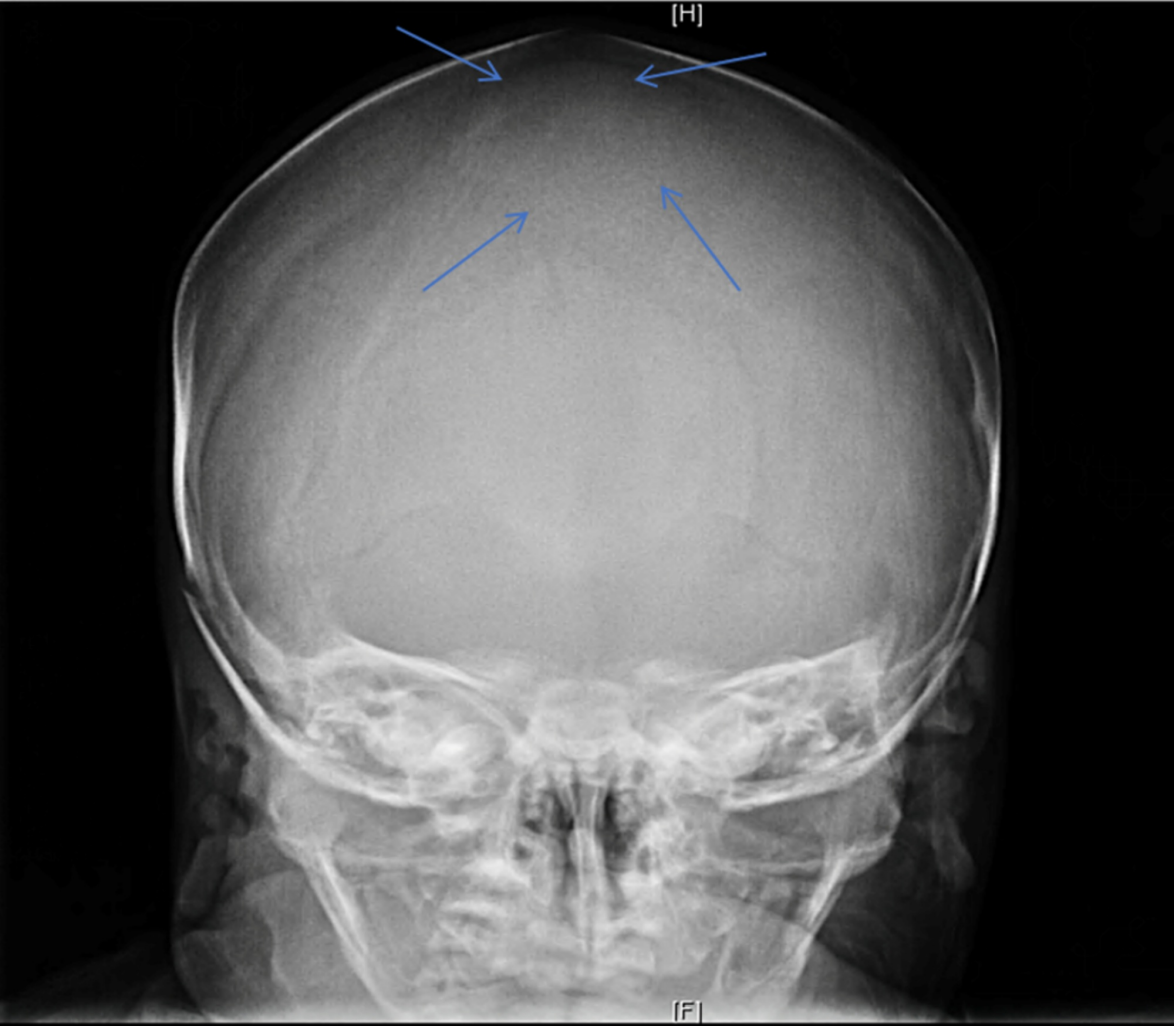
Anterior view in the cranial X-ray series Blue arrows depict the outline of Wormian bone in the anterior fontanelle.
